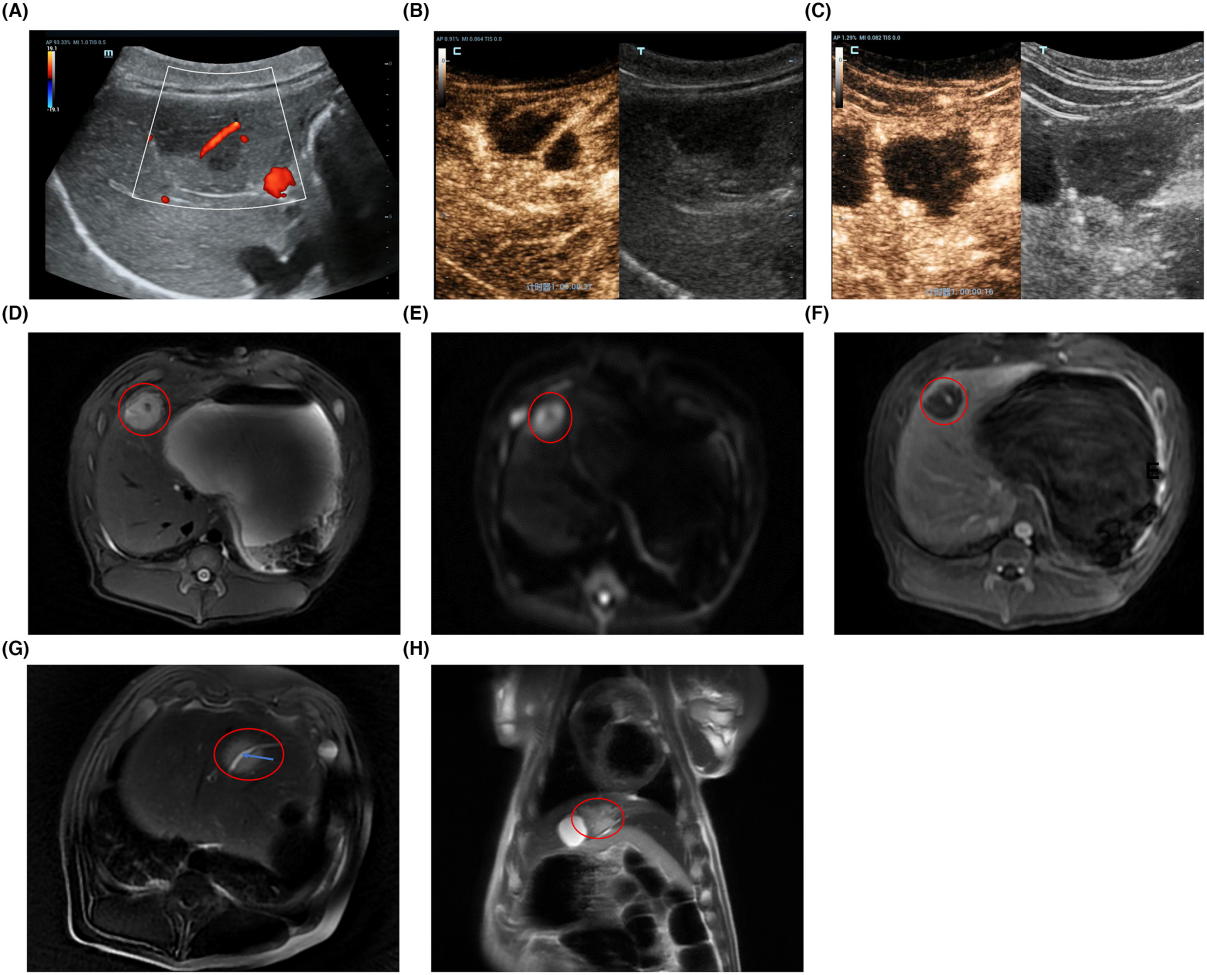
(A) Seven days after H‐FIRE, ultrasound showed that the ablation area appeared as a homogeneously hypoechoic region with a clear boundary. The blood flow in the vessels passing through the ablation region was normal. (B) The CEUS examination performed 7 days after H‐FIRE ablation showed that the ablation region was completely non‐enhanced, but the major blood vessels passing through this region still showed a normal enhancement pattern. (C) The CEUS examination performed 7 days after H‐FIRE ablation showed that the gallbladder wall was intact and the enhancement pattern was normal. (D) Dynamic contrast‐enhanced MRI was performed 7 days after H‐FIRE. The ablation areas still appeared to be an elliptical region with high signal intensity on T2WI, and the boundary was clear. (E) The ablation region was clearly visualized on DWI. (F) The ablation region showed no enhancement during all three phases. (G) The major blood vessel passing through the ablation area was intact. (The blood vessel in ablation area was indicated by a blue arrow). (H) The gallbladder wall adjacent to the ablation area was intact. (The ablation areas were indicated by red circles).
